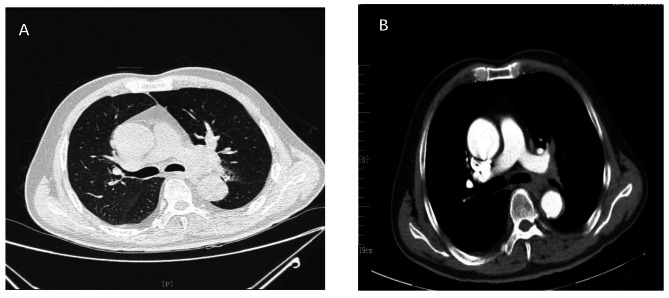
(A-B) Chest computed tomography scan on 2023.09.13. A: Lung window; B: Mediastinum windows
